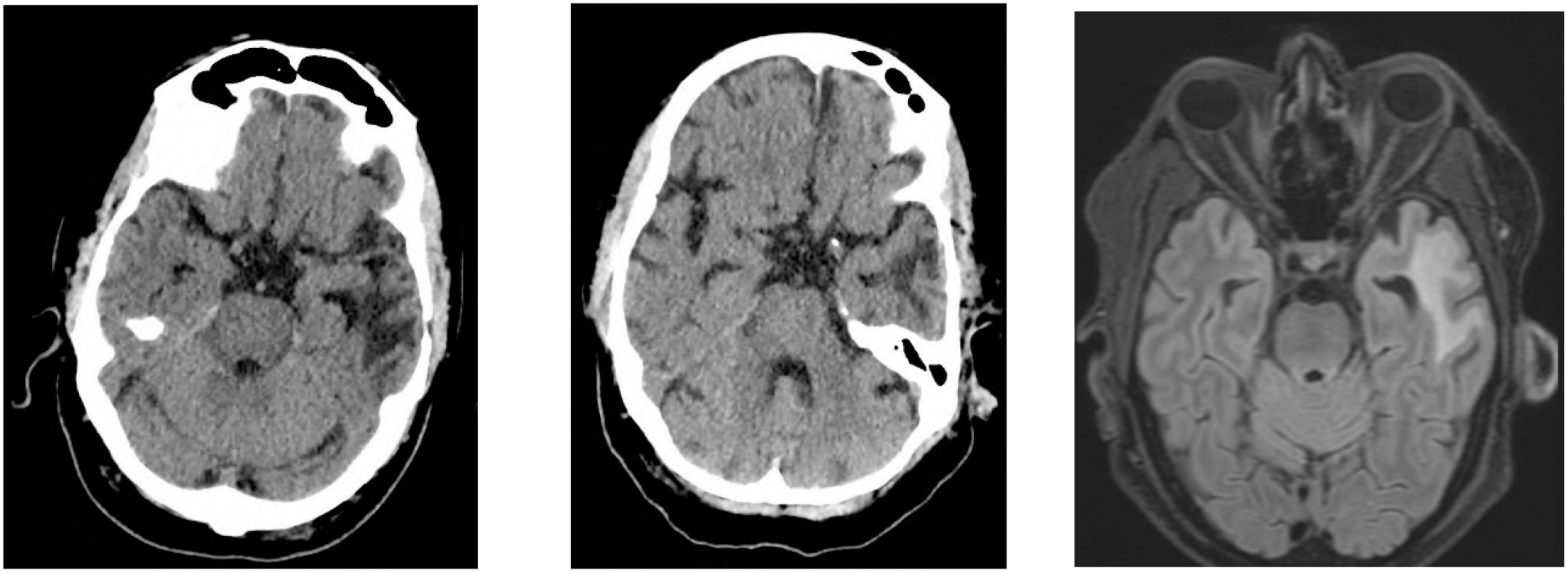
Cerebral imaging until day +28. (A) cCT day +1. (B) cCT day +5. (C) cMRI day +28. (A) On day +1, the patient developed ICANS; CT imaging shows a slightly regressive hypodensity in the right parietal area (right parietal = 2nd lymphoma recurrence). Known defect in the left temporoparietal area following tumour resection (=1st lymphoma recurrence), unchanged. (B) On day +5, due to progressive ICANS grade II-III with aphasia under dexamethasone, a repeat CT scan was performed. There was no indication of right-parietal progression as well as a constant parenchymal defect in the left temporal area due to previous tumour resection, nor were there any signs of intracerebral haemorrhage or early signs of infarction. (C) On day +16, the patient was discharged from the hospital, on day +22 he was readmitted because of MNTs and on day +28, follow-up staging imaging was conducted, which showed no new lymphoma manifestation on MRI.
